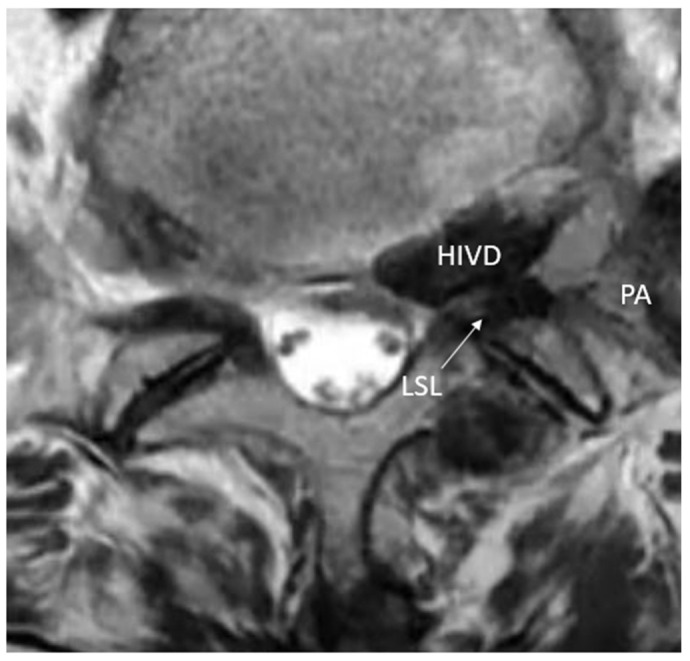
Preoperative axial T2-weighted MRI at the L5-S1 level. The image demonstrates significant entrapment of the left L5 nerve root within the extraforaminal space. It clearly shows compression due to the herniated disc, hypertrophied lumbosacral ligament, and the formation of a pseudoarticulation. HIVD: herniated intervertebral disc; LSL: lumbosacral ligament; PA: pseudoarticulation.
